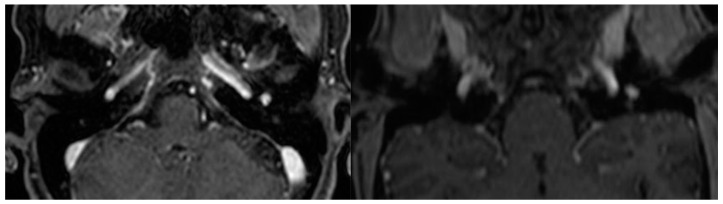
Axial and coronal MRI with gadolinium enhancement sections showing avidly enhancing mass in the middle ear cavity.
